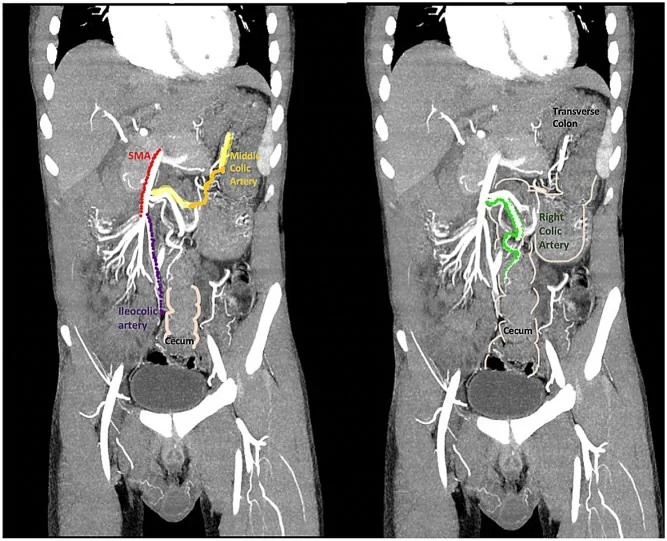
CTA of the abdomen/pelvis with visualization of the SMA and its branches.
